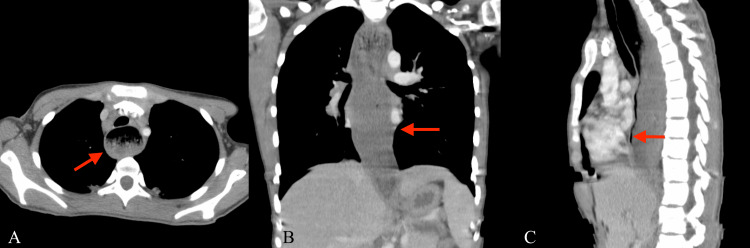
Chest CT with contrast in axial (A), coronal (B), and sagittal (C) views, demonstrating significant distention of the esophagus with fluid and food particulate throughout its course (red arrows) with abrupt tapering at the gastroesophageal junction CT: computed tomography
